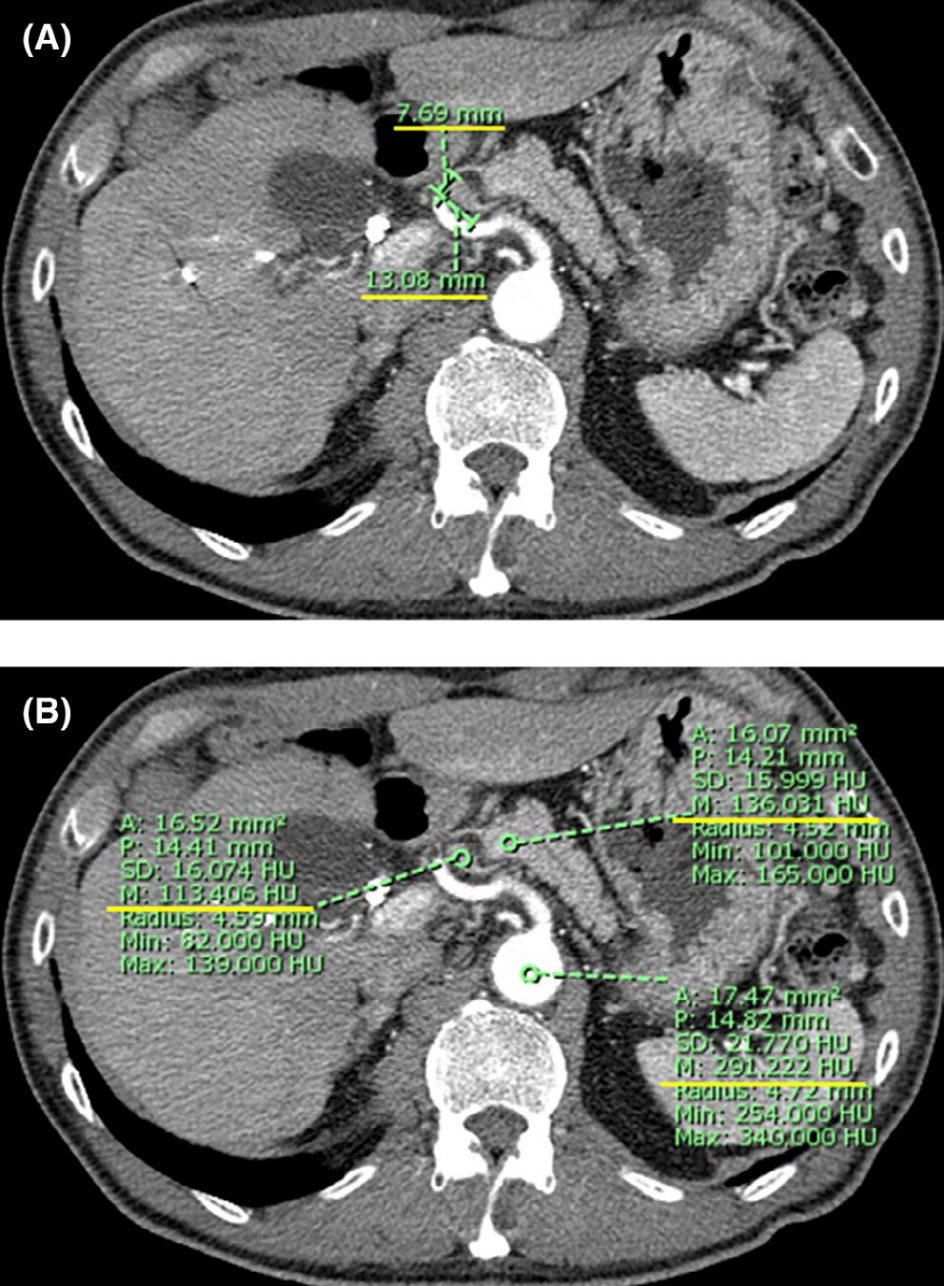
Measurement of N (CHA) parameters on dynamic contrast‐enhanced computed tomography (dCECT). (A) Short‐to‐long axis ratio (SLR): N (CHA) refers to lymph nodes located along the common hepatic artery (CHA). The axial slice of the arterial‐phase dCECT showing the maximum long‐axis diameter of the node; the short‐axis diameter is measured perpendicular to the long axis. SLR was defined as the short‐axis diameter divided by the long‐axis diameter. In this example, 7.69/13.08 = 0.59. This parameter represents the roundness of the lymph node. (B) Hounsfield unit (HU) ratios—HU (LN‐aorta) and HU (LN/pancreas): A circular region of interest (ROI) was positioned around the N (CHA) node to calculate its mean HU value. The mean HU values of the aorta and pancreatic parenchyma are measured on the same slice, and the ratios HU (LN/aorta) and HU (LN/pancreas) were calculated. In this example, HU (LN/aorta) = 113.406/291.222 = 0.39, and HU (LN/pancreas) = 113.406/136.031 = 0.83. These parameters reflect the contrast enhancement of the lymph node.
